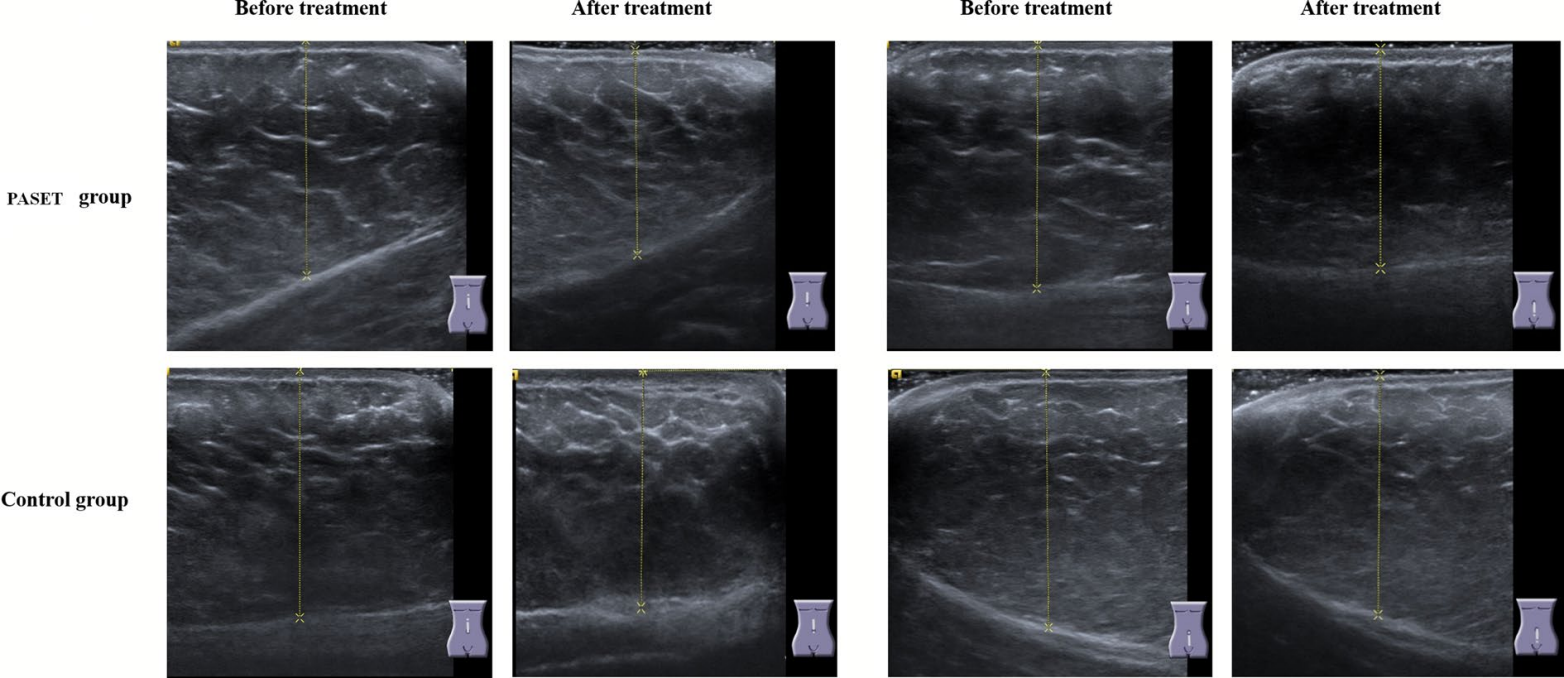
The measurement of abdominal subcutaneous fat tissue’s thickness by using B-model ultrasound. A 15-MHz linear transducer was placed perpendicular to the skin surface. Subcutaneous fat thickness (mm) was measured as the perpendicular distance from the skin surface to the upper border of the adipose/muscle interface
